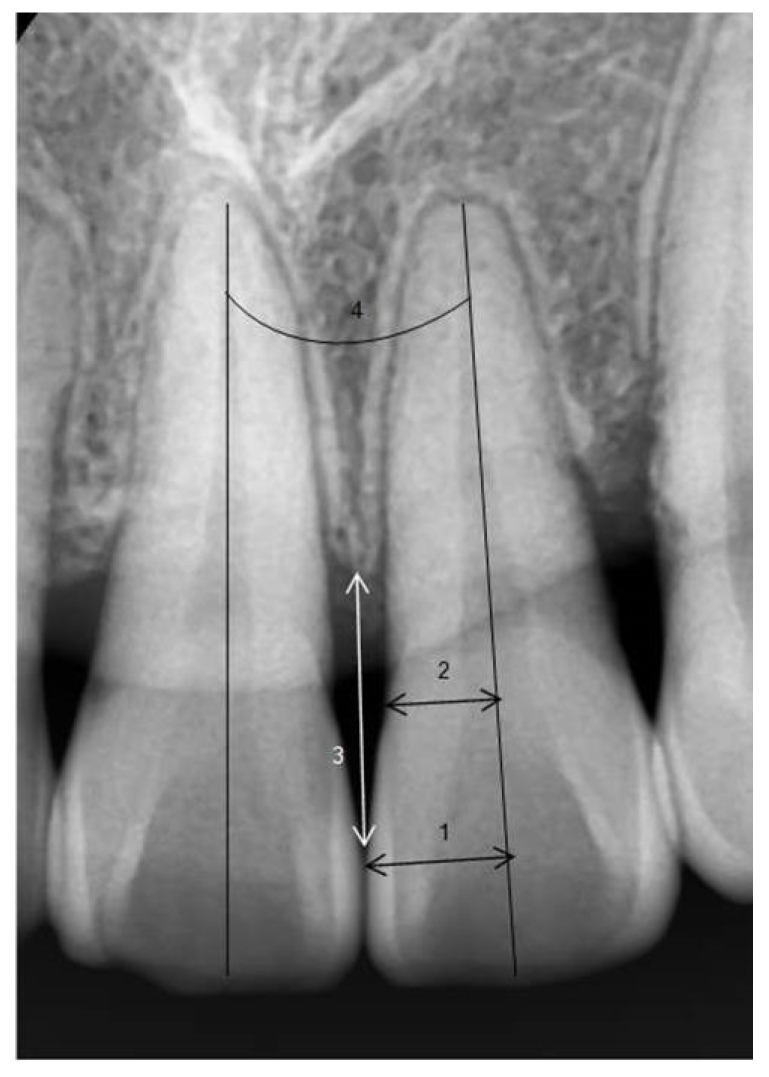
Periapical radiographic measurements. 1, perpendicular distance from the mesial interproximal contact to the tooth long axis; 2, perpendicular distance from the mesial CEJ to the tooth long axis; 3, distance of alveolar bone–interproximal contact; 4, post-treatment root angulation classification of black triangle according to Jemt Index.
